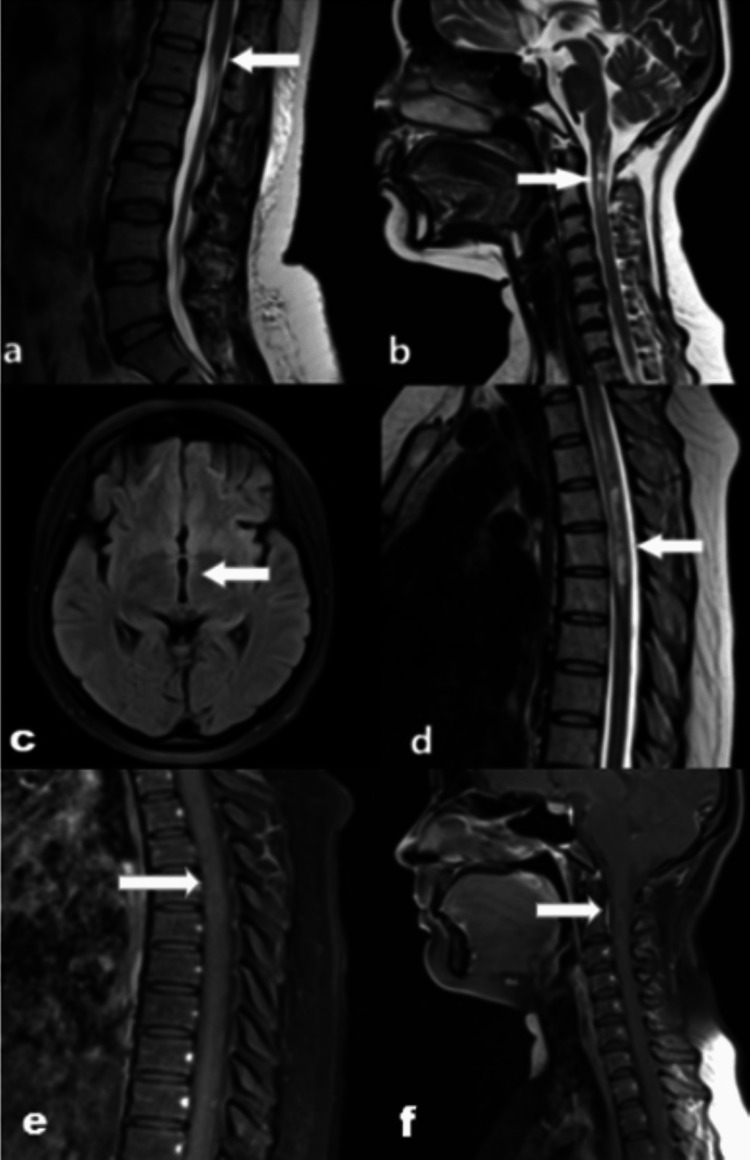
Previous magnetic resonance imaging of the spine and brain of the same patient done one year ago 3a: T2-weighted sagittal image of the spine at the level of the conus medullaris showing no abnormal signal intensity (white arrow). 3b: T2-weighted sagittal image showing short-segment hyperintensities in the cervicomedullary junction and upper cervical spinal cord (white arrow). 3c: T2-weighted axial image of the brain showing no abnormal signal intensities in the periventricular region of the third ventricle (white arrow). 3d: T2-weighted sagittal image of the spine showing long-segment hyperintensities in the dorsal spinal cord (white arrow). 3e: Post-contrast T1-weighted sagittal image of the spine showing no significant enhancement in dorsal spinal cord lesions (white arrow). 3f: Post-contrast T1-weighted sagittal image of the spine showing no significant enhancement in cervical spinal cord lesions (white arrow).
